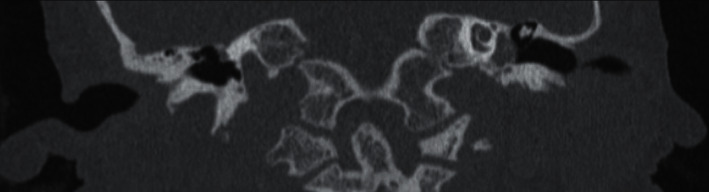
CT coronal view. Left mesotympanic and hypotympanic mass extending into the infracochlear space. Visible sharp.
